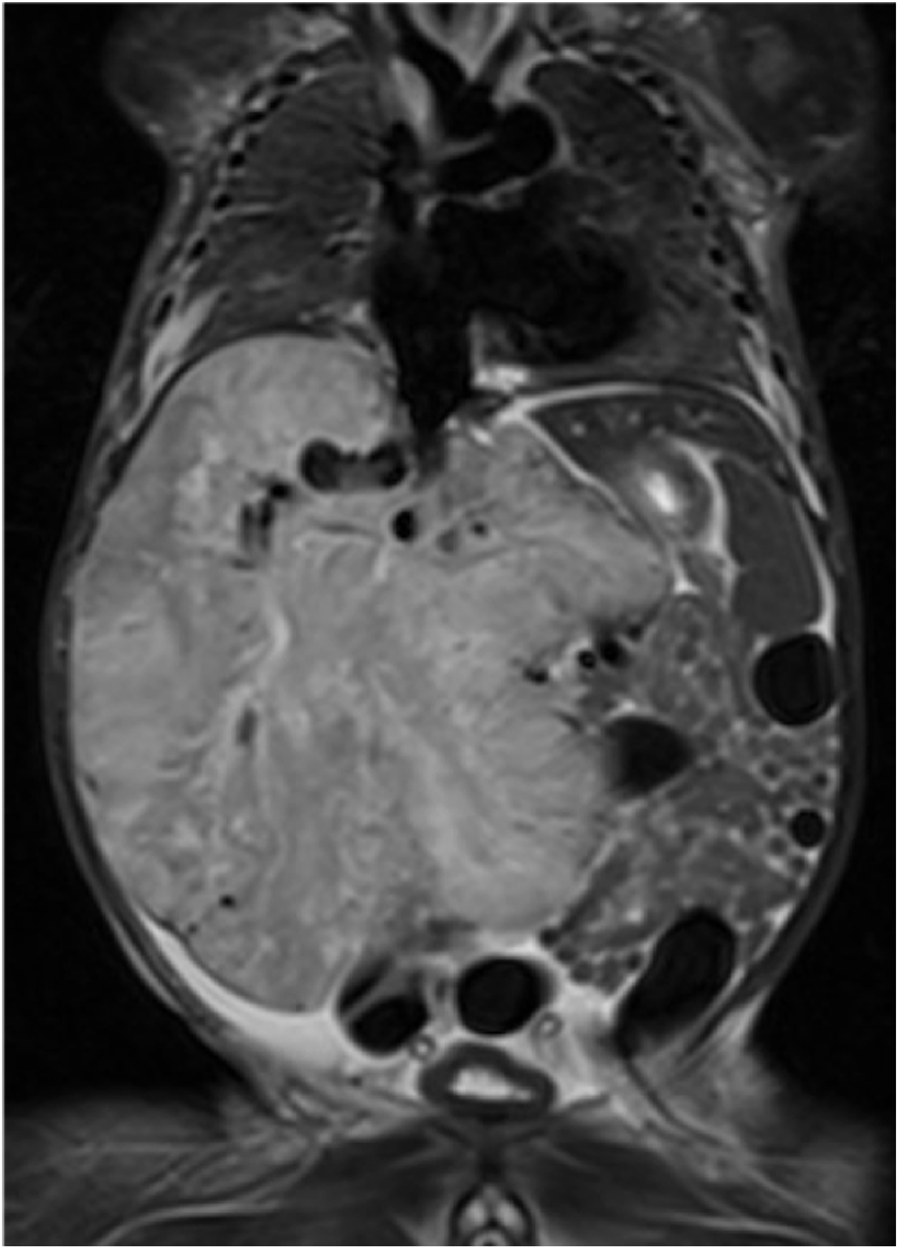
Coronal T2 weighted MR image, showing a large rapidly-involuting congenital haemangioma (RICH) occupying almost all of the liver in a 3-day old male. The lesion caused splinting of the diaphragm, high output cardiac failure and abdominal compartment syndrome
